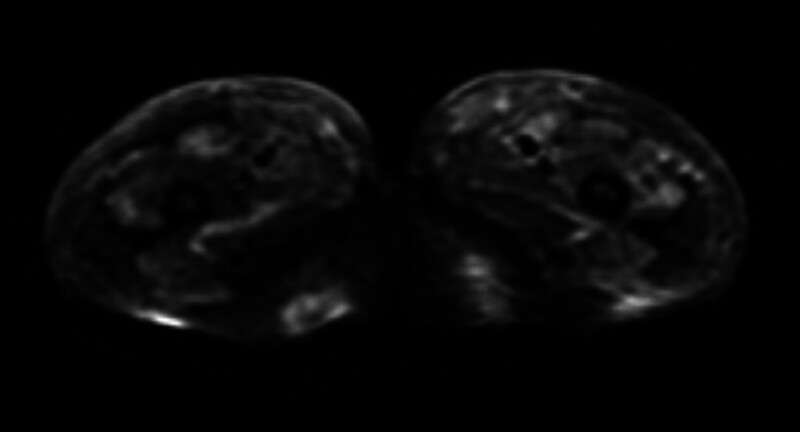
Hyperintense signals were observed in both quadriceps femoris muscles on magnetic resonance imaging, including diffusion-weighted imaging with background suppression and short-tau inversion recovery.
